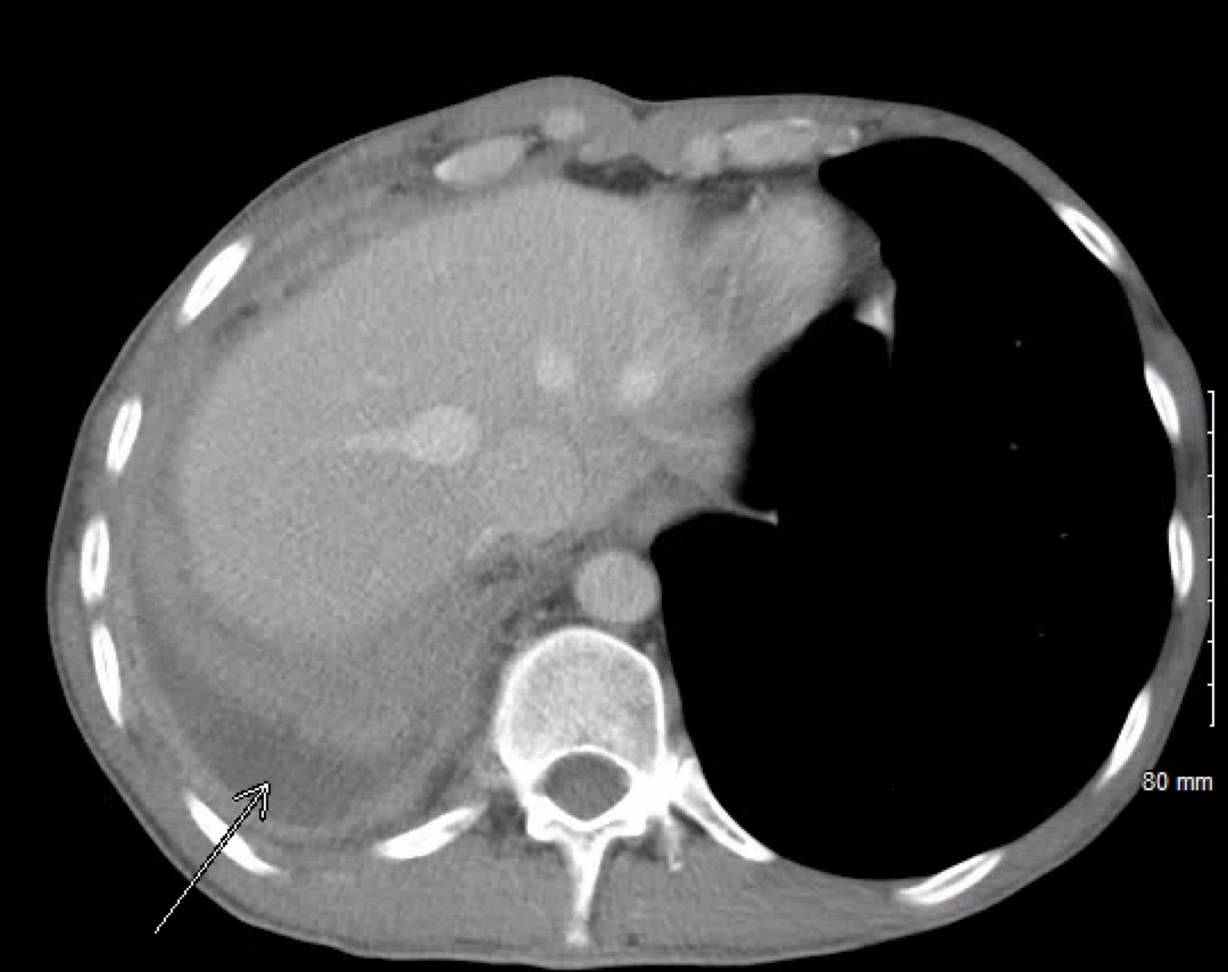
CT chest showing loculated pleural effusion on right side indicated by arrow
